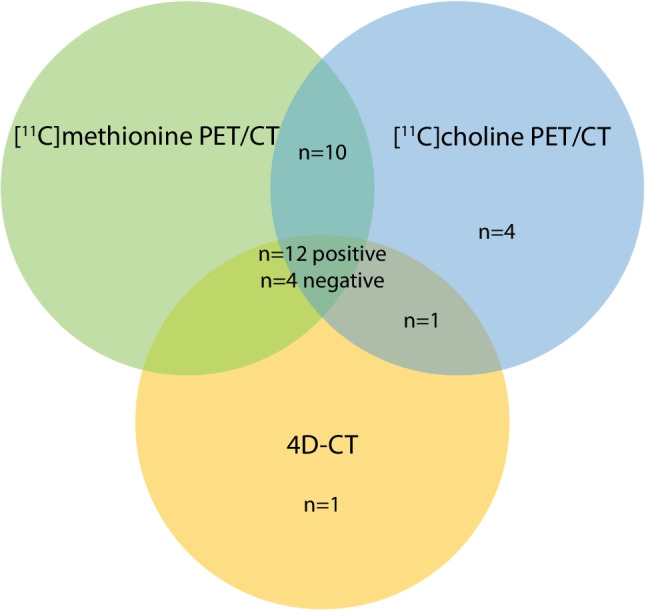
Overlap between scan interpretation of [^11^C]methionine PET/CT, [^11^C]choline PET/CT, and 4D-CT with blinded scan interpretation (patient-based, *n* = 32). In *n* = 12 patients, all scans were positive, and in *n* = 4 patients, all scans were negative
